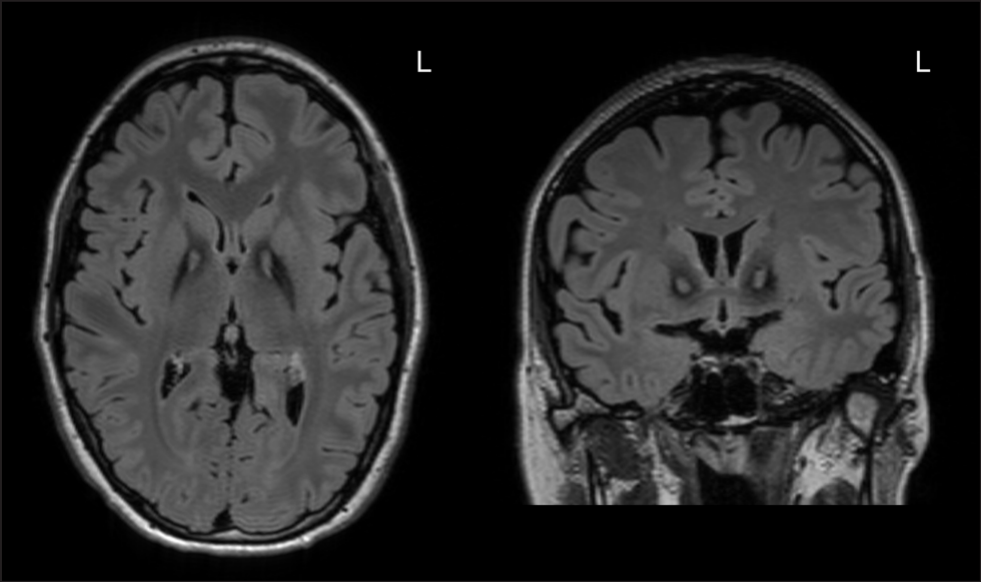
Axial (left) and coronal (right) T2-FLAIR brain MRI showed bilateral hypointensity of the globus pallidus with anterior medial hyperintensity, consistent with the “eye of the tiger” sign. L = left.
